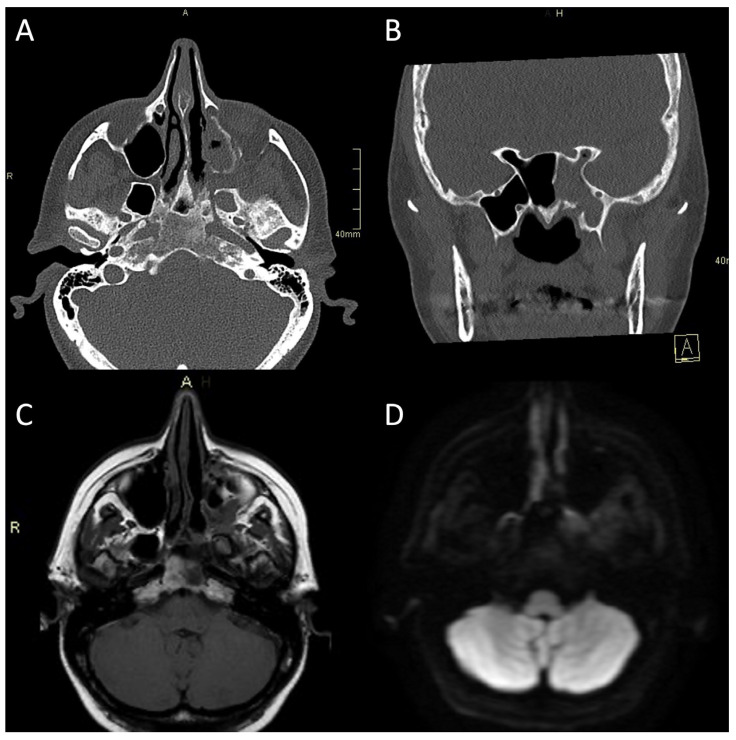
CT and MRI of a patient with bacterial atypical skull-base osteomyelitis. (A) Axial CT bone algorithm reconstruction showing expanded left pterygopalatine fossa, opacified ipsilateral maxillary, and sphenoid sinus, with mixed erosive and hyperostotic bony changes. (B) Coronal CT bone algorithm reconstruction showing asymmetric expansion and destruction of the left Vidian canal; A—anterior. (C) Axial MRI T1 showing clival bone marrow oedema. (D) Axial MRI DWI showing increased signal in the left pterygoid base, pterygopalatine fossa, and infratemporal fossa. CT: computerised tomography; MRI: magnetic resonance imaging; CE: contrast-enhanced; DWI: diffusion-weighted imaging.
